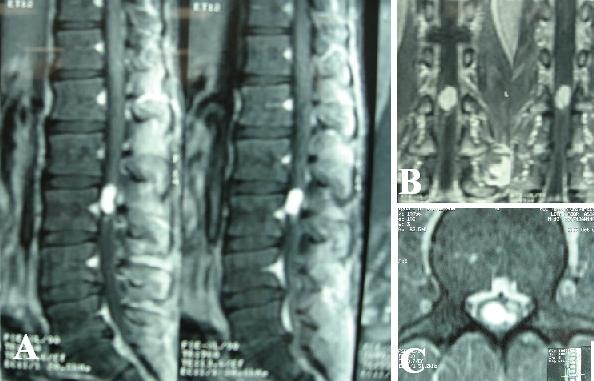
Post-gadolinium enhancement revealed in sagital (A), coronal (B), and axial (C) magnetic resonance images of this lesion.
